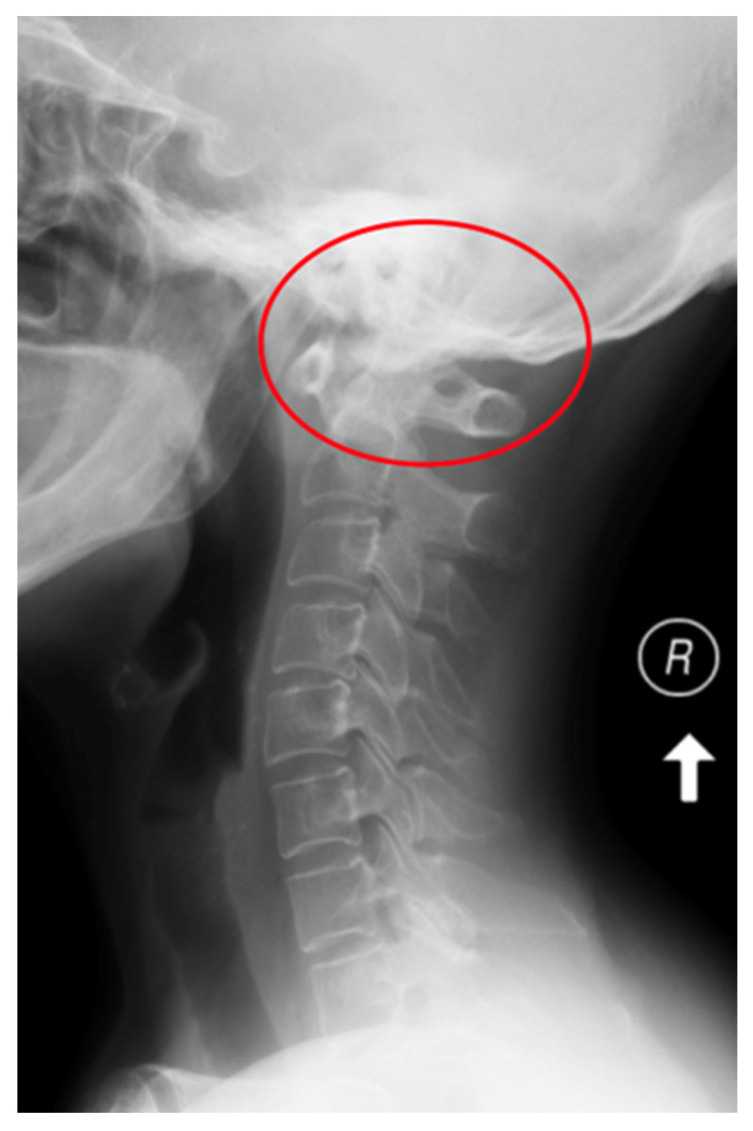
An X-ray of the cervical spine (lateral view) of a 65-year-old female patient with RA and AAS instability.
